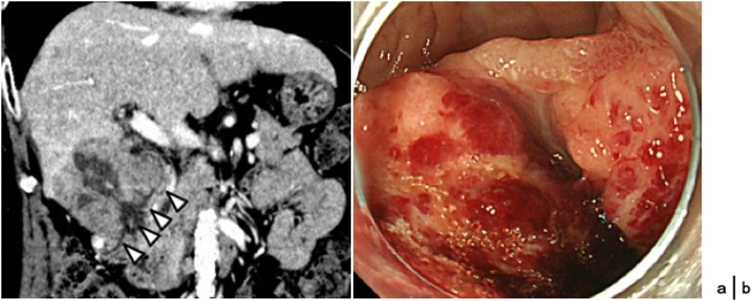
(a) Abdominal enhanced CT scan showed the tumor in the liver segment 5, invading the first and second portion of the duodenum (arrowheads). (b) An upper GI endoscopy revealed an ulcerative lesion on the posterior wall of the duodenal bulb.
